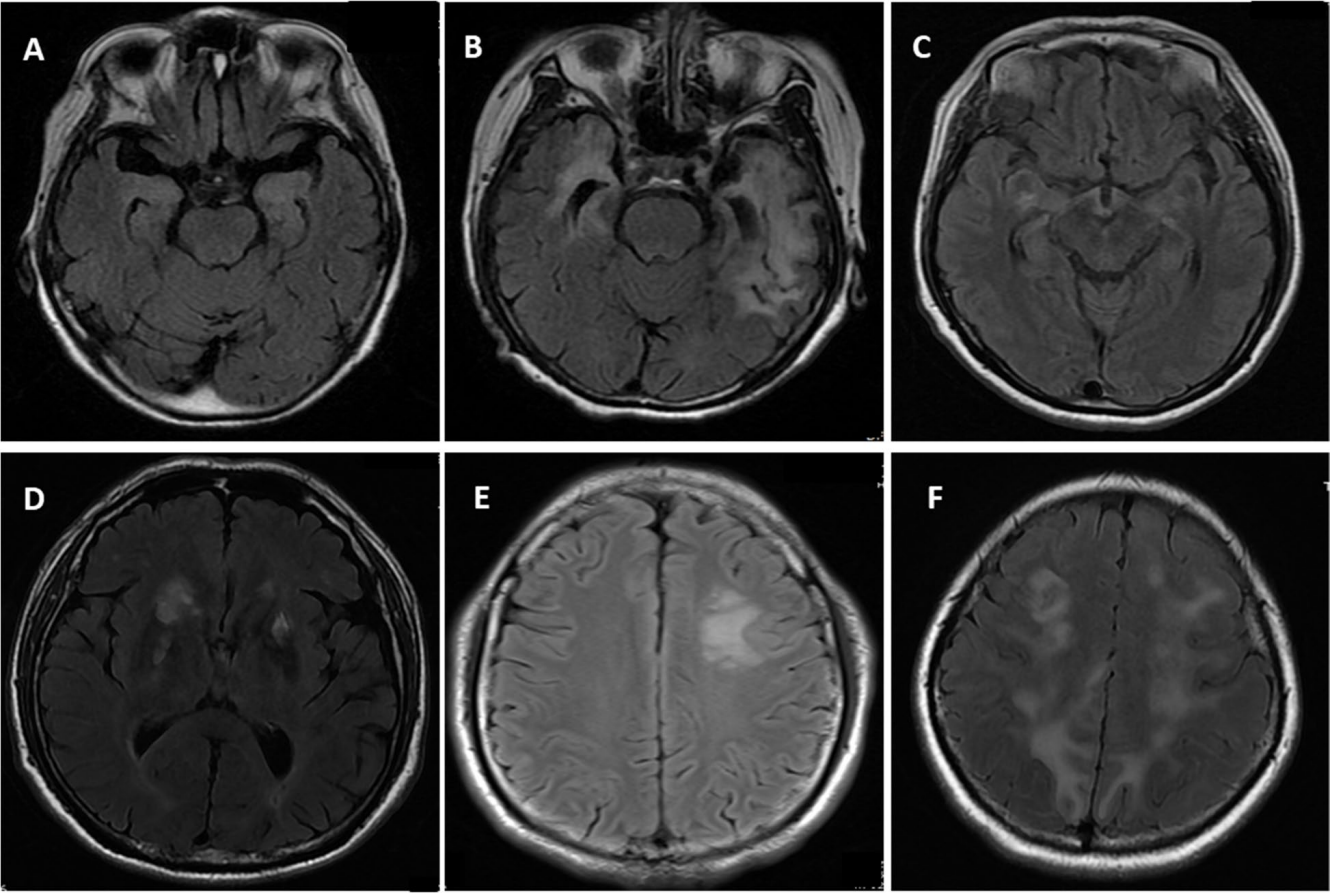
Brain T2/ FLAIR axial MRI specific changes of the patients with DPPX antibodies. A Mild increased T2/FLAIR signals of the bilateral hippocampus (Patient 1); B T2/FLAIR increased signals of the bilateral temporal lobe and hippocampus (Patient 9); C Mild T2/FLAIR increased signals of the bilateral amygdala (Patient 2); D Increased T2/FLAIR signals in the right basal ganglia and thalamus (Patient 7); E T2/FLAIR increased signals of the left centrum semiovale (Patient 4); F Extensive abnormal T2/FLAIR signals in the bilateral frontal lobe and parietal lobe (Patient 6)
